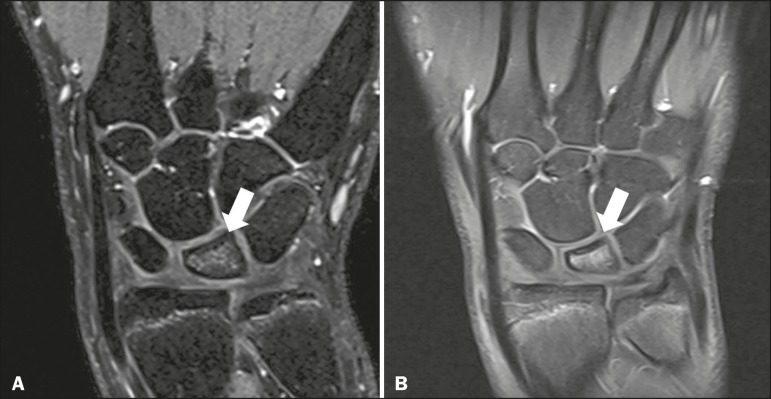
Contrast-enhanced T1- and T2-weighted MRI sequences, both
